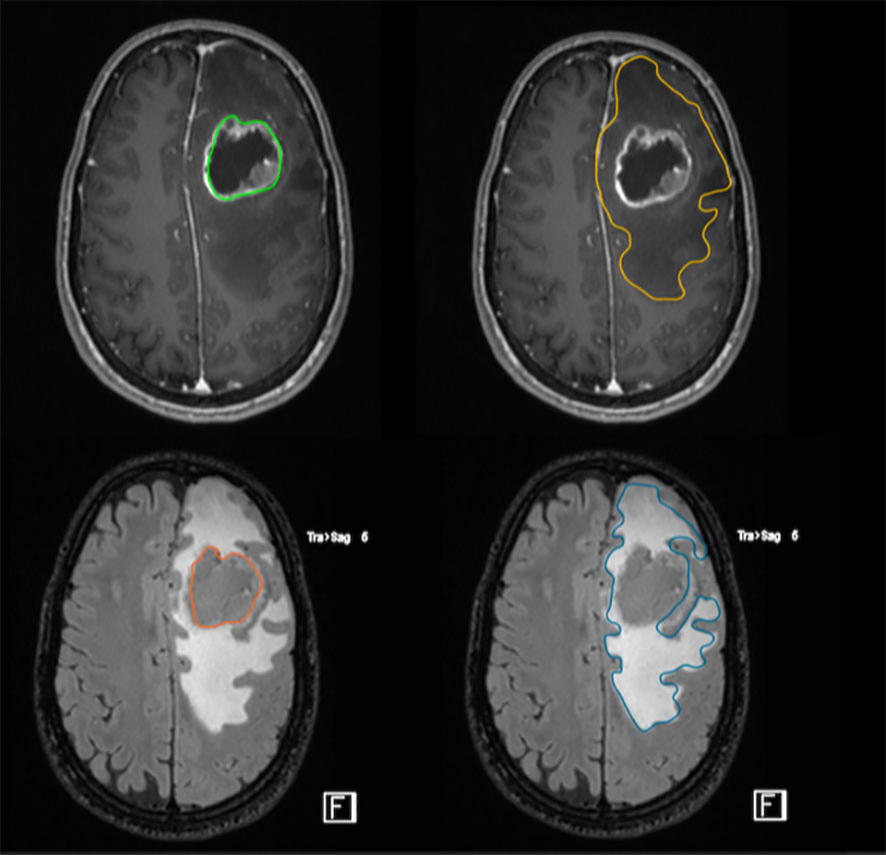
FLAIR-high lesions showing peritumoral brain zone in glioblastoma. The size of the peritumoral edema is one index to predict the degree of tumor infiltration through imaging. The surgical resection of FLAIR-high lesions is important to improve postoperative outcome.
